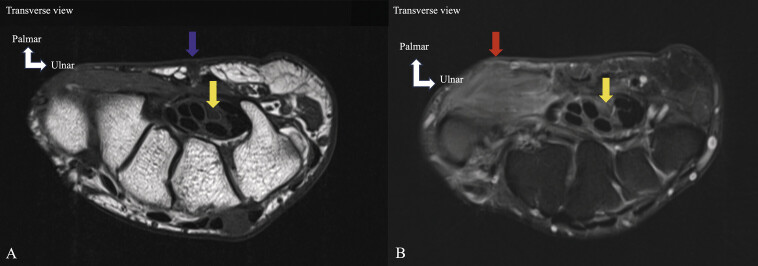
Recurrent carpal tunnel syndrome. MRI (
